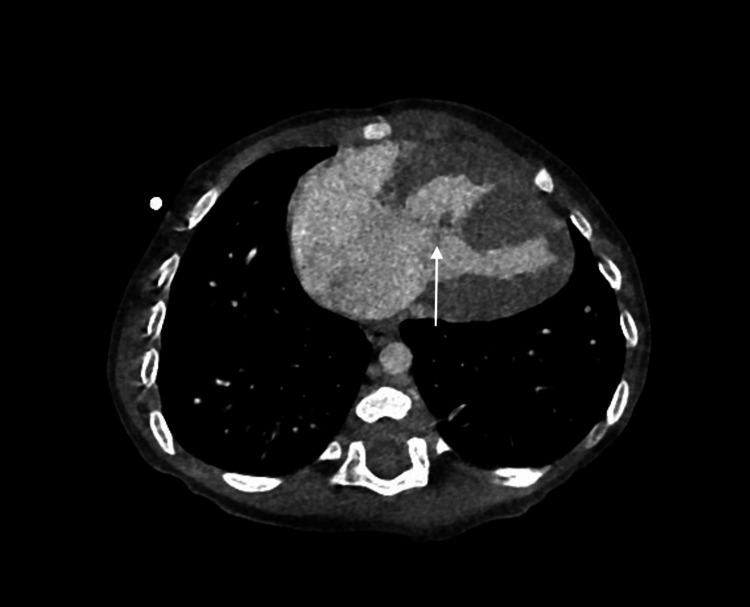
Axial contrast-enhanced computed tomography demonstrating a transitional atrioventricular septal defect The white arrow indicates absence of the atrioventricular septum, with a common atrioventricular junction.
